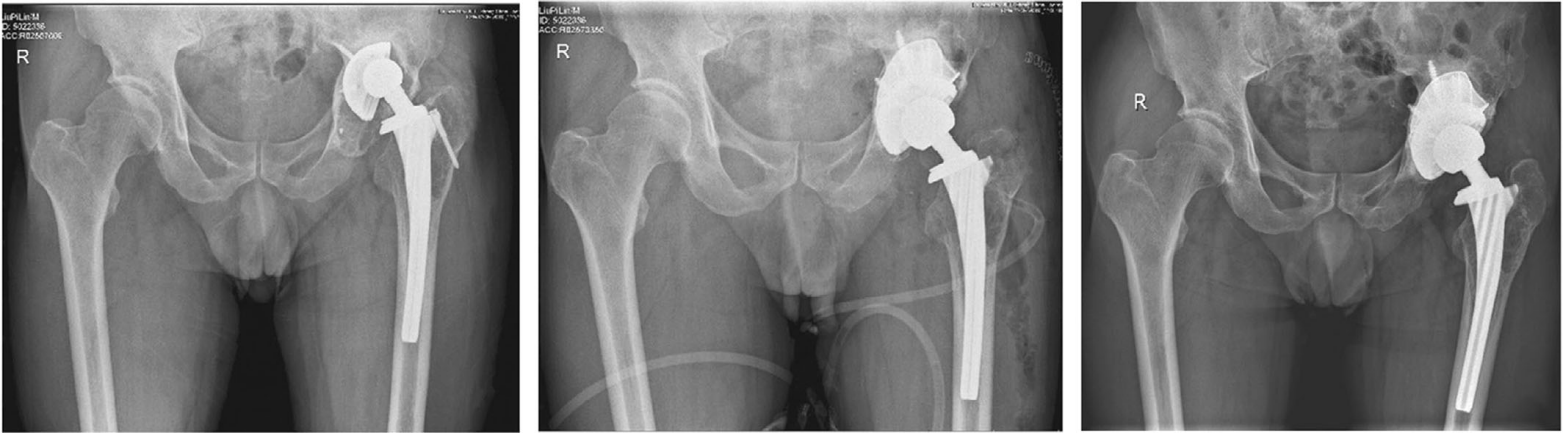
Radiographs showing an example of the use of a 3D printed tantalum augment (left hip) for the reconstruction of a Paprosky type IIB defect: (A) preoperative, (B) postoperative, and (C) last follow‐up anteroposterior (AP) radiographs at 3 years.^53^
